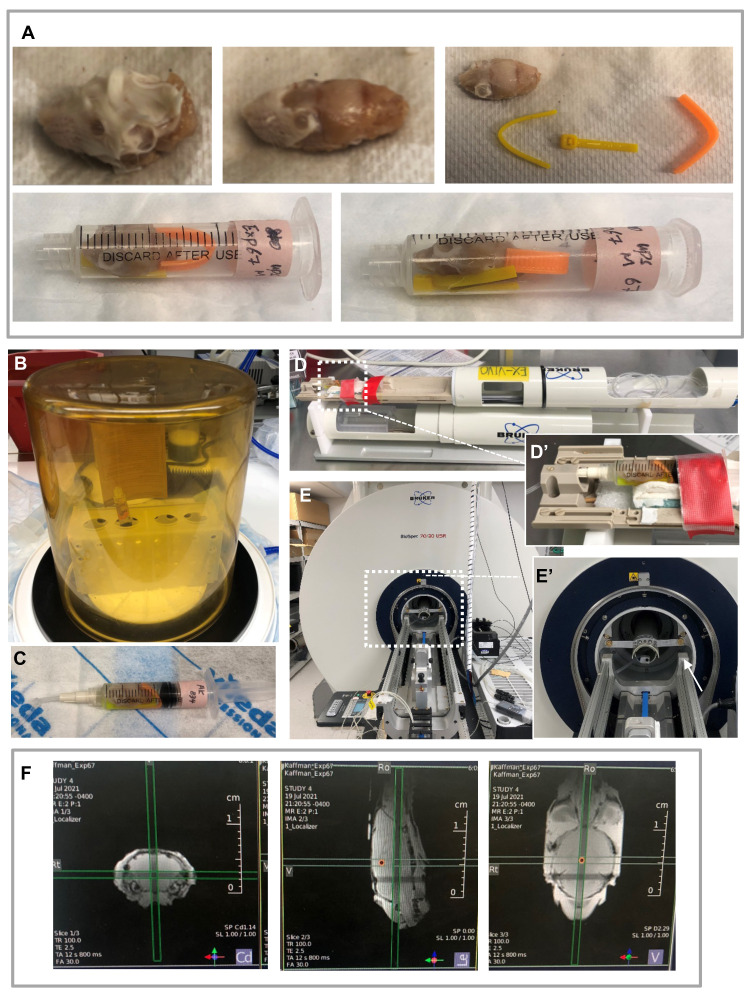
Preparation for MRI. A. Sample preparation: Remove the tissues outside of the skull carefully without damaging the eyeballs (top panel). Place the brain in a 5ml syringe with small pieces of zip-ties to fix its position (bottom panel). B-C. Remove air from the syringe: Connect the syringe to a loosely tied vacutainer filled with Fomblin^®^ (shown in C) and place in the vacuum chamber (shown in B). Remove the vacutainer and turn on the vacuum for 30 min to remove air bubbles. Push out the remaining air after vacuum and seal the top by tightening the vacutainer. D. Place the sample in a manufacturer-made sample holder designed for the cryogenic probe. D’. A zoom-in view of the sample. E. Insert the sample holder into the magnet bore of the magnet. E’. A zoom-in view of the holder (indicated by the white arrow). F. Three orthogonal plane images acquired using the Localizer protocol on a 7 Tesla Bruker preclinical MRI system.
